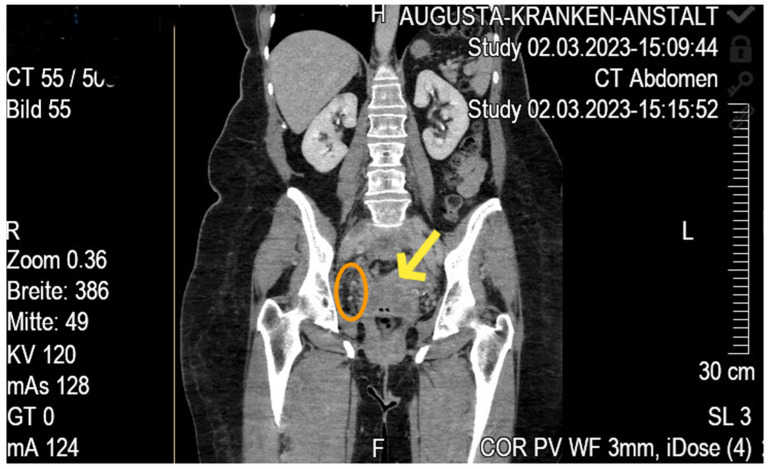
Contrast-enhanced axial CT of the abdomen and pelvis. Yellow arrow = 7 cm hyper dense presacral mass compressing right external iliac vein; dashed circle = cluster of collateral veins in the inguinal region indicating venous outflow obstruction.
